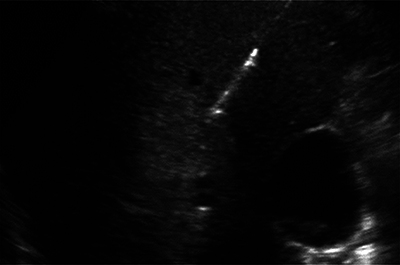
Ultrasound images of the hepatic lesion biopsy: Round hyperechoic formation with a posterior acoustic enhancement.
